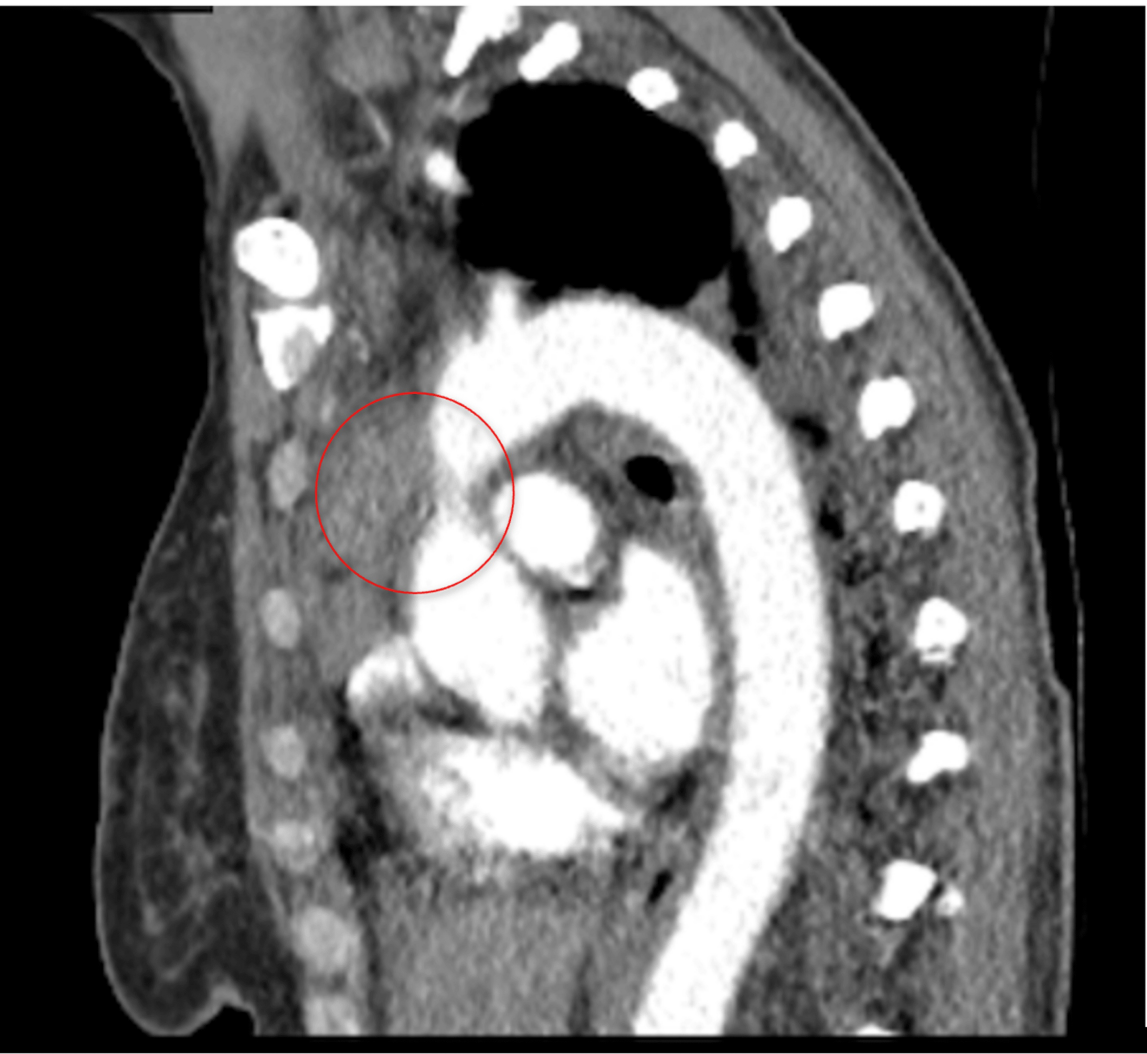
Sagittal computed tomography angiogram showing further progression of the infiltrative mediastinal mass, now extending into the pericardium and encasing the aortic arch as circled in red.
